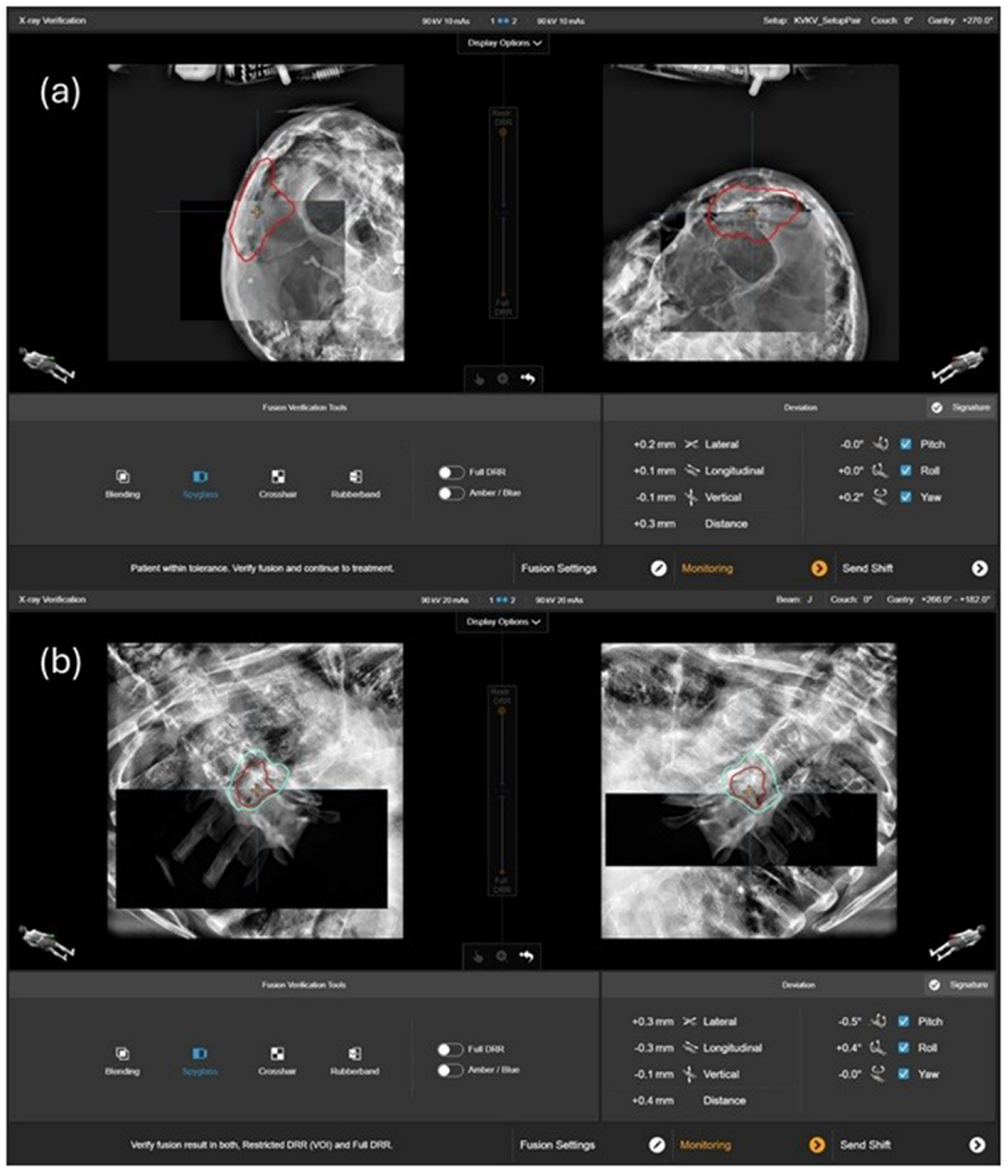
X-ray verification of stereoscopic images for a cranial (a) and a spinal (b) case.
